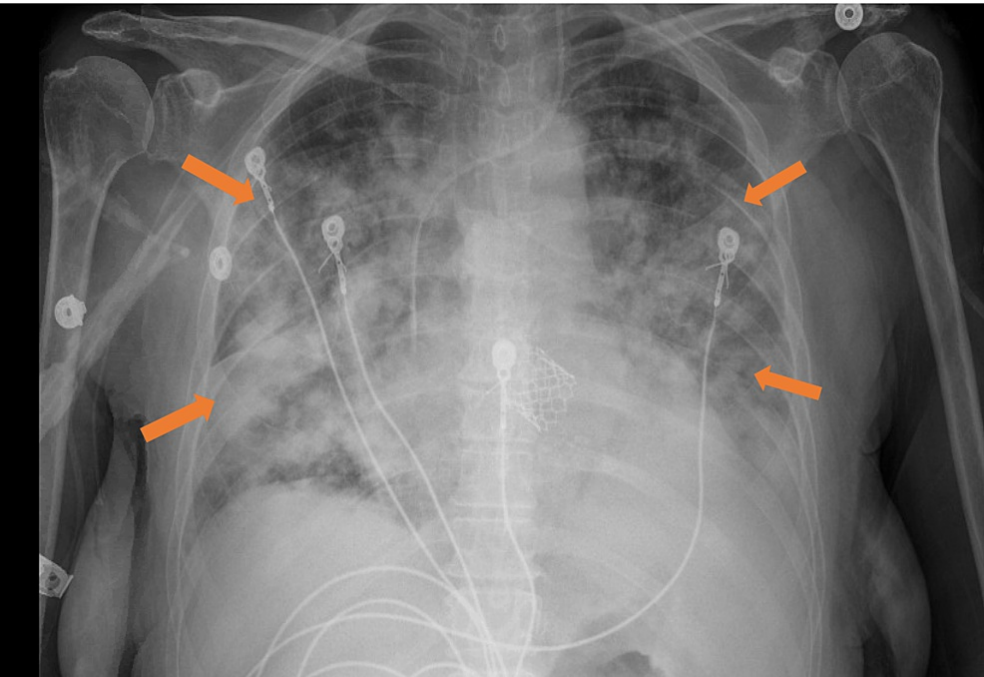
Chest x-ray 7 hours post-transfusion - Bilateral diffuse alveolar infiltrates (indicated by all the arrows)
